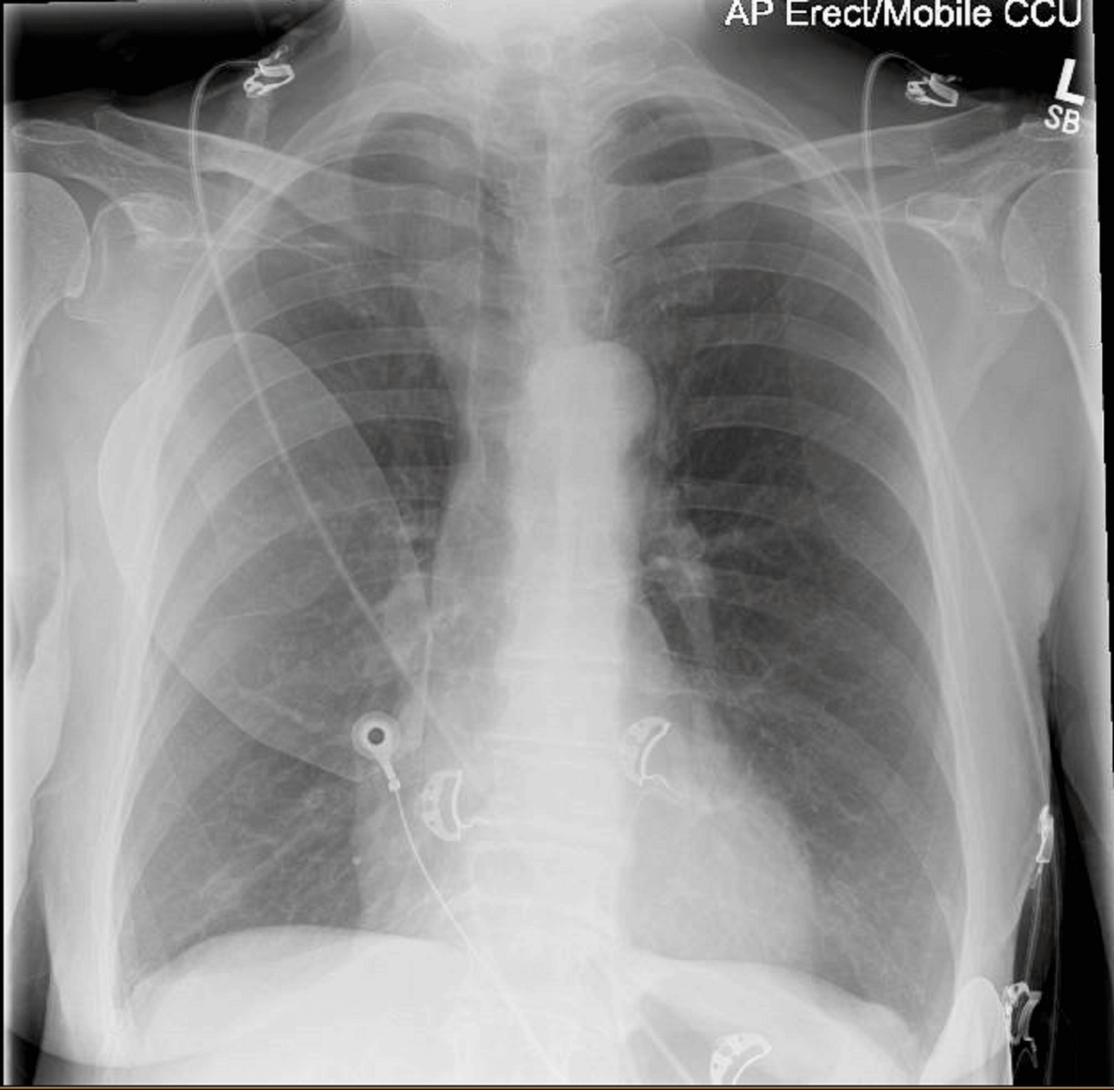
Chest radiograph Chest X-ray did not show any pulmonary edema
